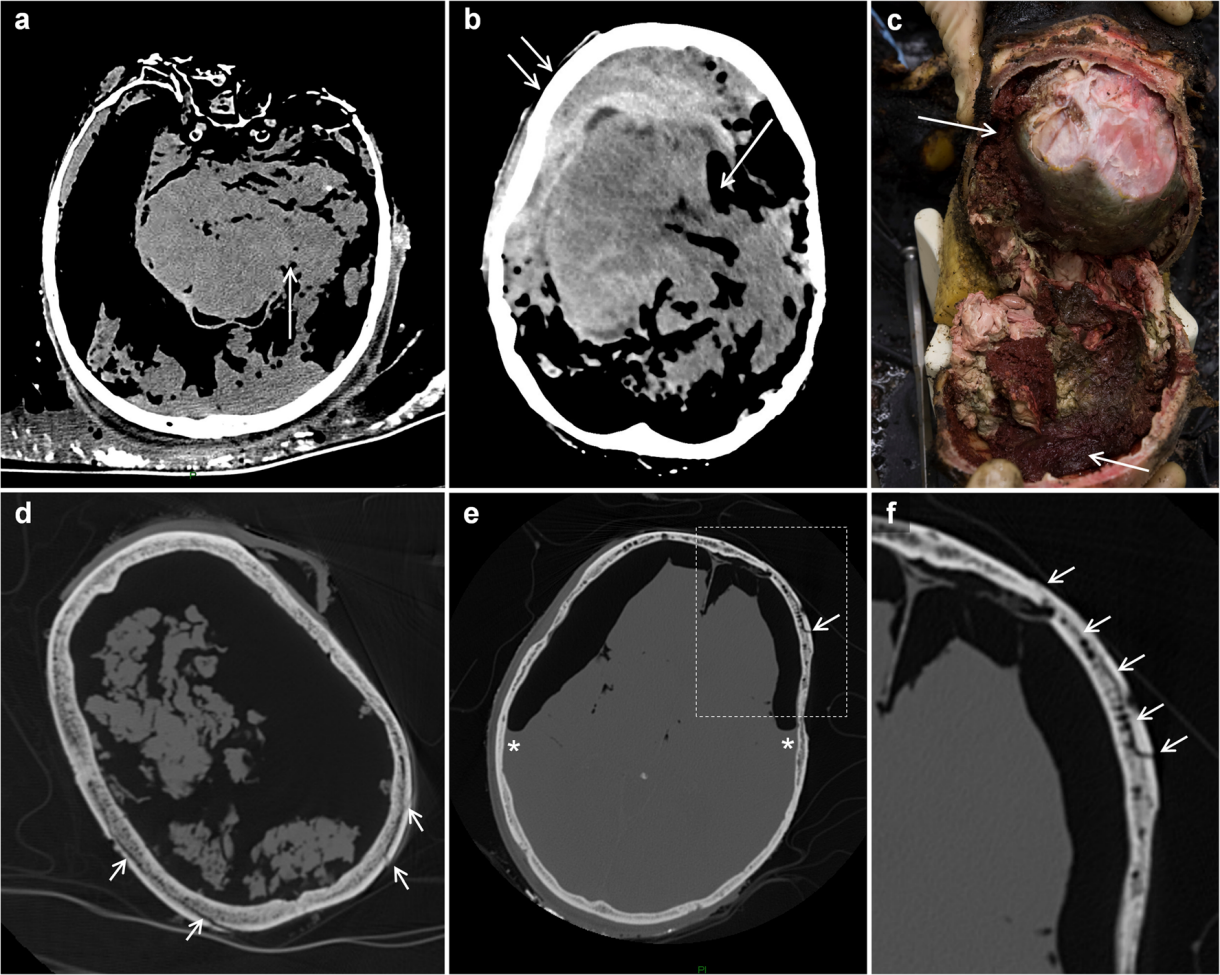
Typical post-mortem CT findings in the head exposed to fire. a, b Axial images. In both cases, a dural tear and herniated brain tissue can be seen (*single arrow*). The case in (b) shows also an intracranial *heat haematoma* (*double arrow*). c Picture of an opened skull with *heat haematoma* (*arrows*) during autopsy. d, e Axial images. In both cases, heat fractures can be seen in the outer table (*arrows*) on locations where all soft tissue are burned away, resulting in separation of inner and outer table (*split diploë sign*). The case in (e) shows also a bilateral intracranial *heat haematoma*; *air-fluid level. f Enlarged section of (e). The *arrows* indicate the *split diploë sign*
